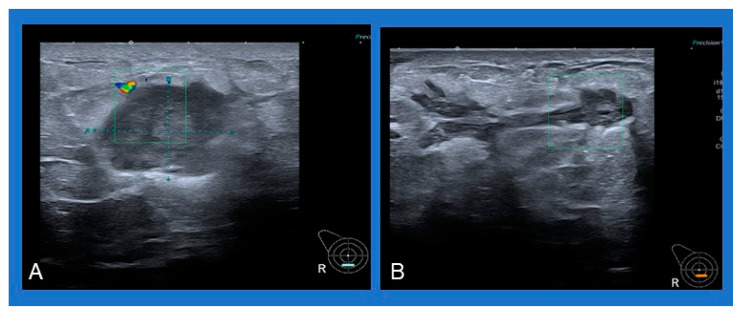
Typical sonographic appearance of IGM: (A) heterogeneous mass with ill-defined margins, (B) multiple fluid collections with floating debris.
